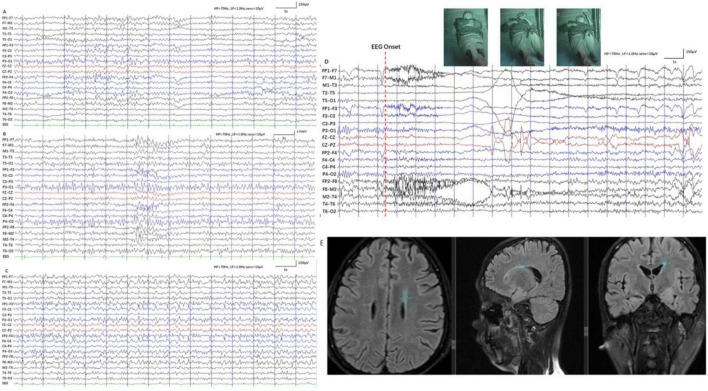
EEG and Imaging data of patient 1. (A–C) EEG of patient 1 at 20 years old, 23 years old and 24 years old. Background of EEG slows down at 20 years old (A), paroxysmal slow waves lasting for less than 3 s at 23 years old (B), lasting for 20–30 s at 24 years old (C). Progressive slowing of the electroencephalographic (EEG) background is prominent. (D) EEG of patient 1 at 24 years old. The ictal EEG was characterized by right cerebral focus with diffused electrodecremental event, subsequently evolving into bilateral hemispheres. (E) MRI images of patient 1 at 23 years. MRI: revealed abnormal signal in right-sided centrum semiovale.
